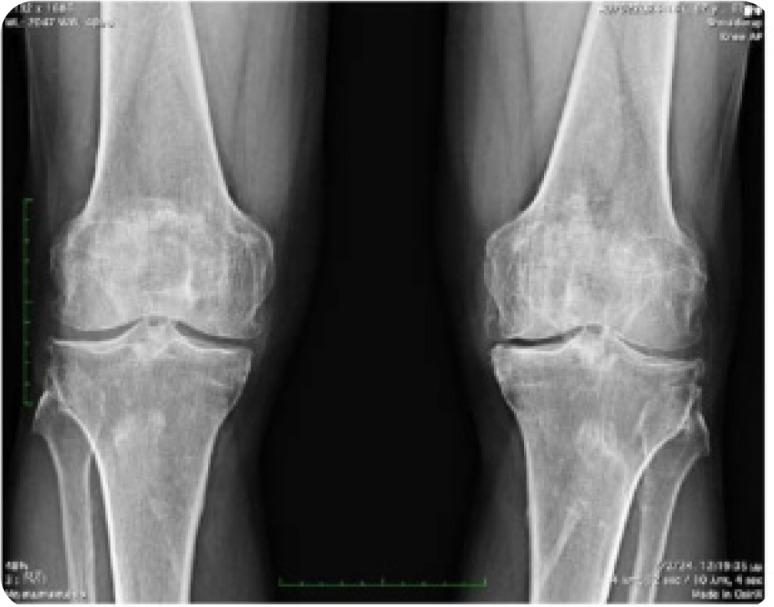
Radiographs of the knees showed extensive degenerative changes with the presence of osteophytes and tricompartmental joint space narrowing.
